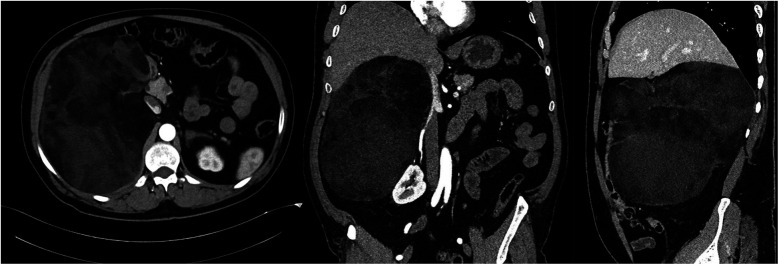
Computed tomography images of a giant right adrenal fatty mass.
